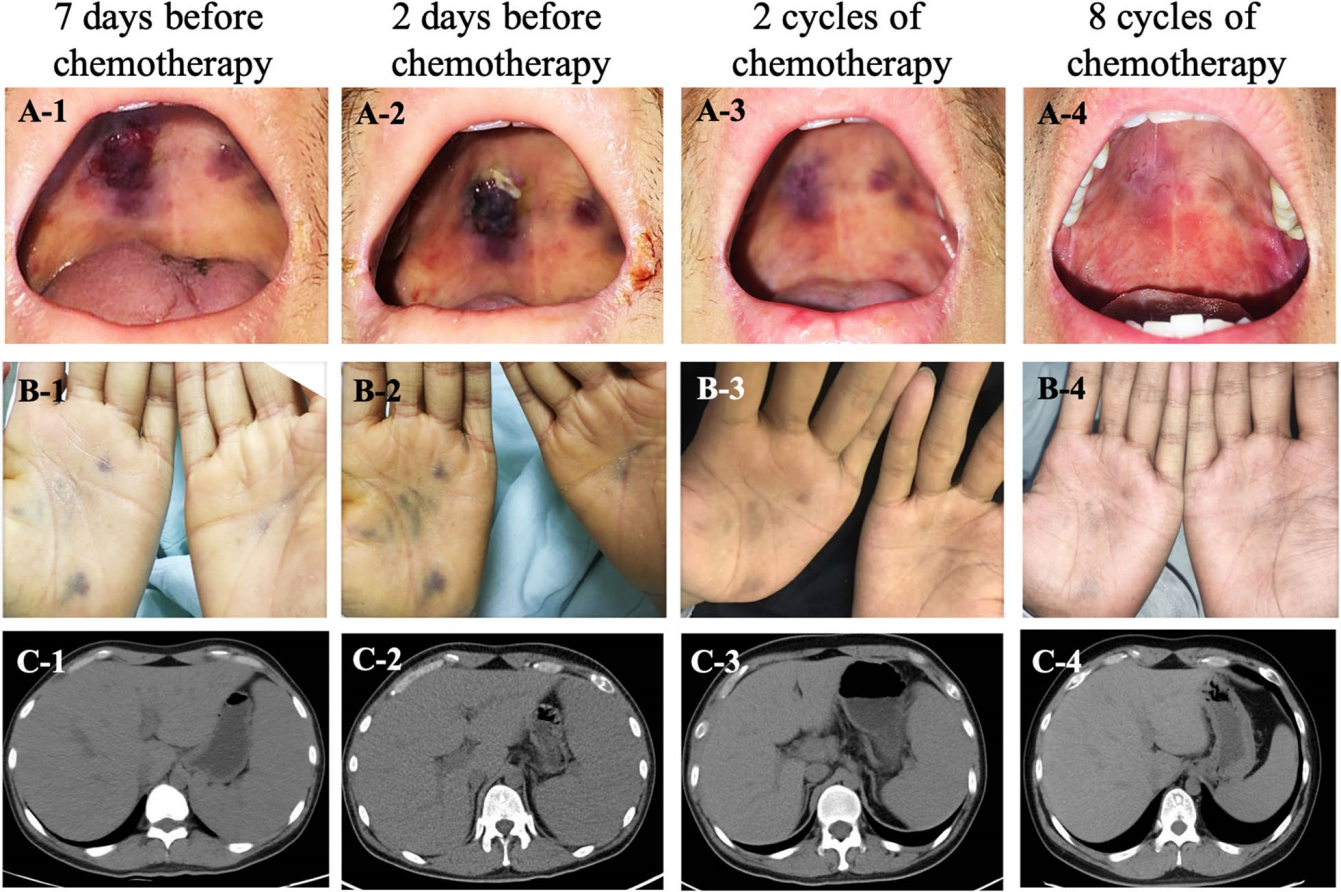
The changes in lesions and spleen before and after chemotherapy. The lesions on oral and palmar surfaces increased both in size and number before chemotherapy (a-1, a-2, b-1, b-2), but they faded away after chemotherapy (a-3, a-4, b-3, b-4). The abdominal CT scans showed splenomegaly before chemotherapy (c-1, c-2), but the size of the spleen decreased to normal (c-3, c-4) after chemotherapy
